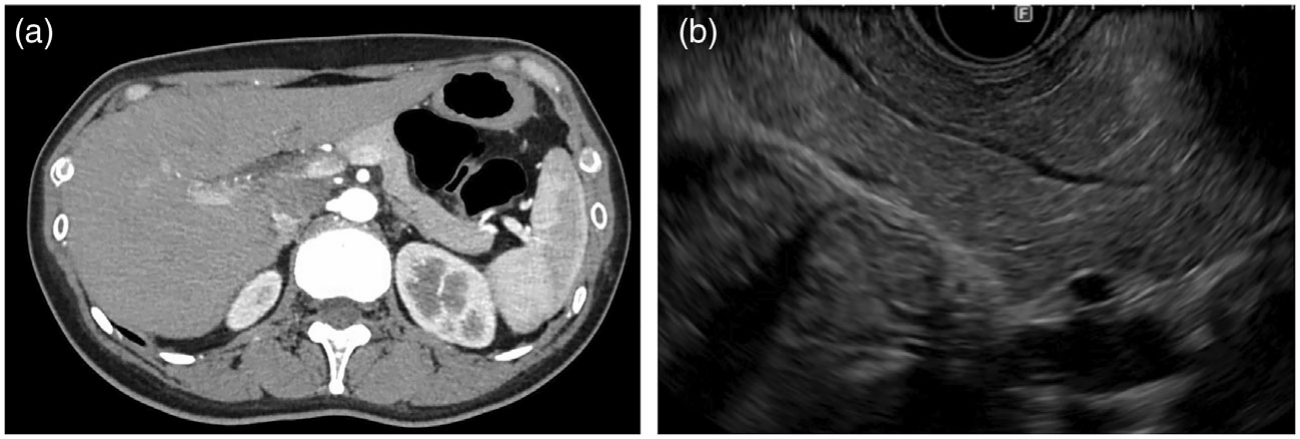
(a) Dynamic computed tomography could not detect the tumor. (b) The tumor could not be detected by B‐mode endoscopic ultrasound.
